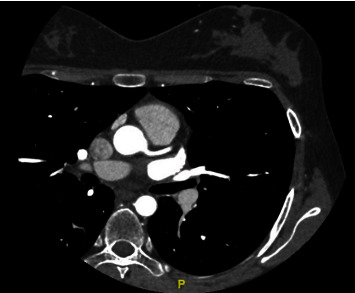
CTA of the coronary vessels demonstrates a patient left main and proximal segment of the left anterior descending branching off of the aorta.
